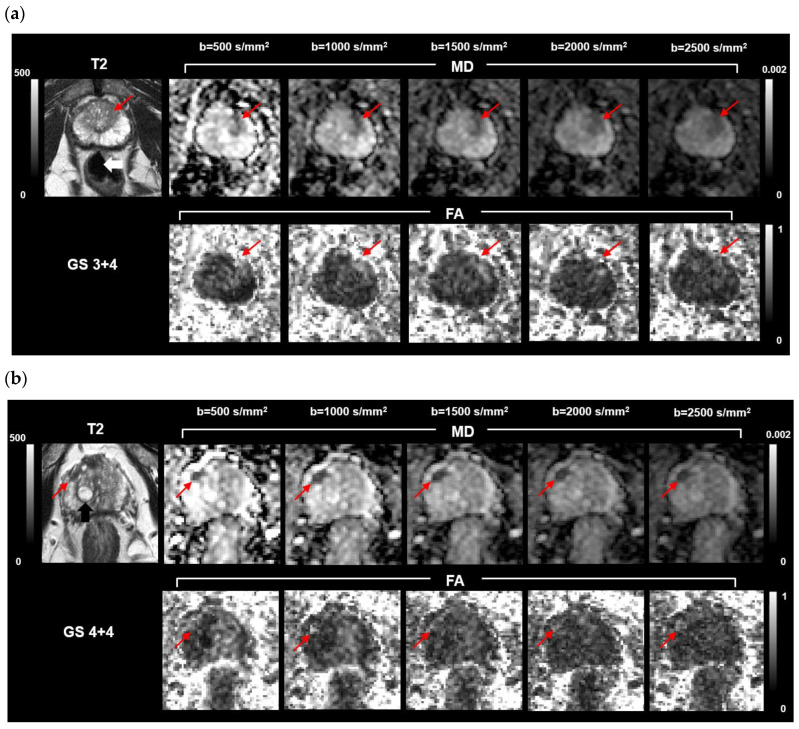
Parametric maps of two patients whose targeted biopsy revealed lesions in the left central gland with GS = 3 + 4 (a), and in the right peripheral zone with GS = 4 + 4 (b). Axial T2-weighted maps, MD, and FA maps are displayed for increasing b-values. The color bars indicate the intensity of T2-weighted maps in arbitrary units (left) and MD intensity expressed in mm^2^/s, and FA, unitless (right). The lesions (red arrows) appear hypointense in both the T2 and MD maps, and slightly hyperintense in the FA maps. A cyst full of liquid appears hyperintense in the T2 maps (black arrow); the rectum is also visible (white arrow).
